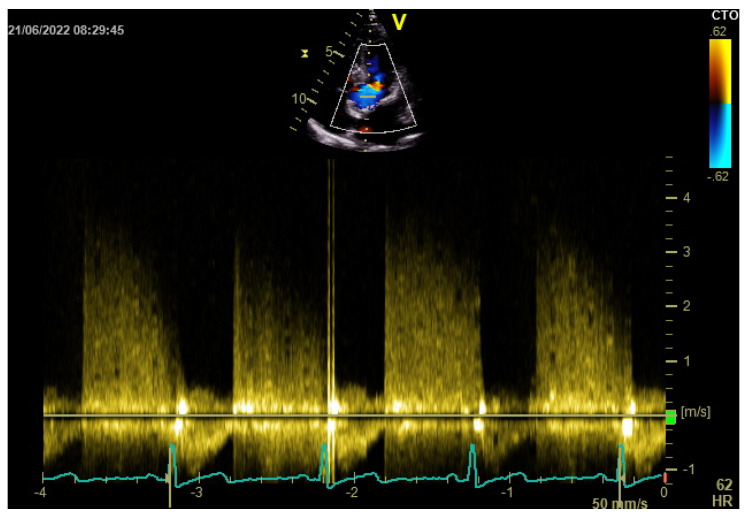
Transthoracic echocardiography: color Doppler echocardiographic images in apical five chamber view and continuous wave Doppler spectrum from the left ventricular outflow tract showed aortic regurgitation flow. The pressure halftime (PHT) was 0.412 seconds.
